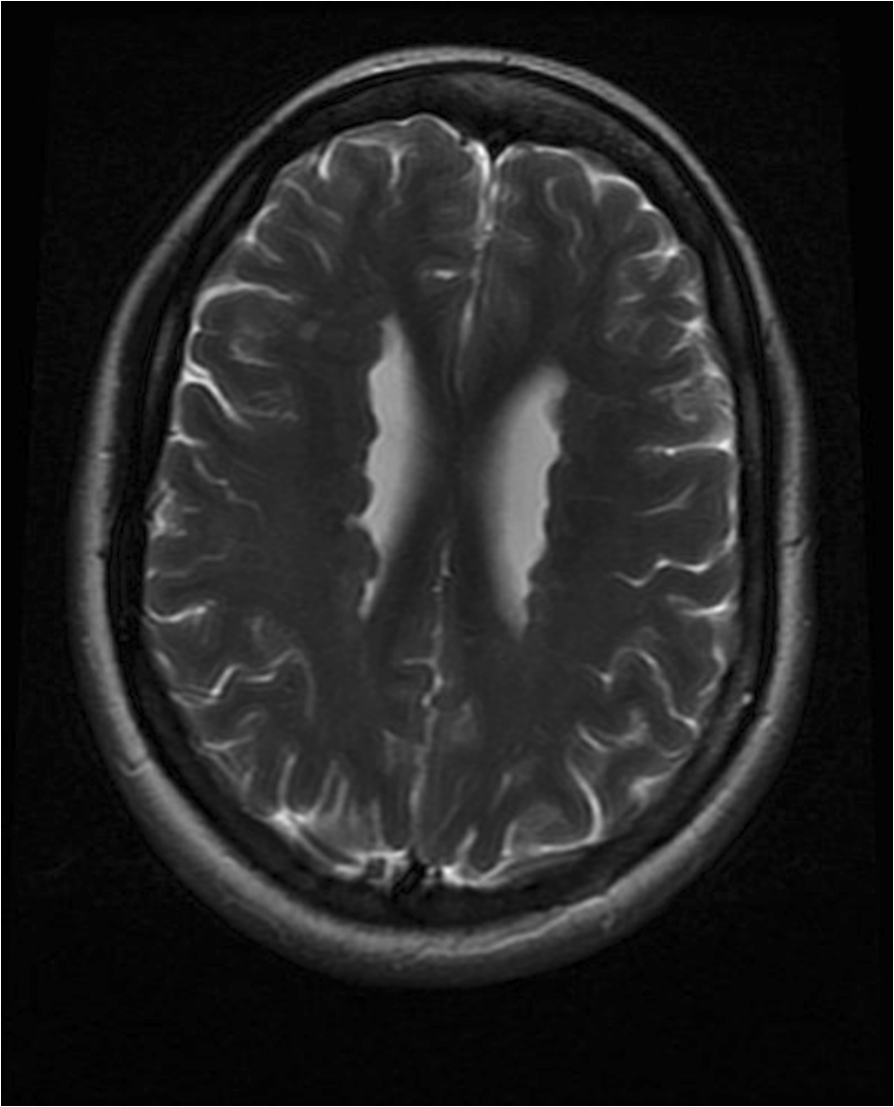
Brain MRI of the index patient showing PNH.
